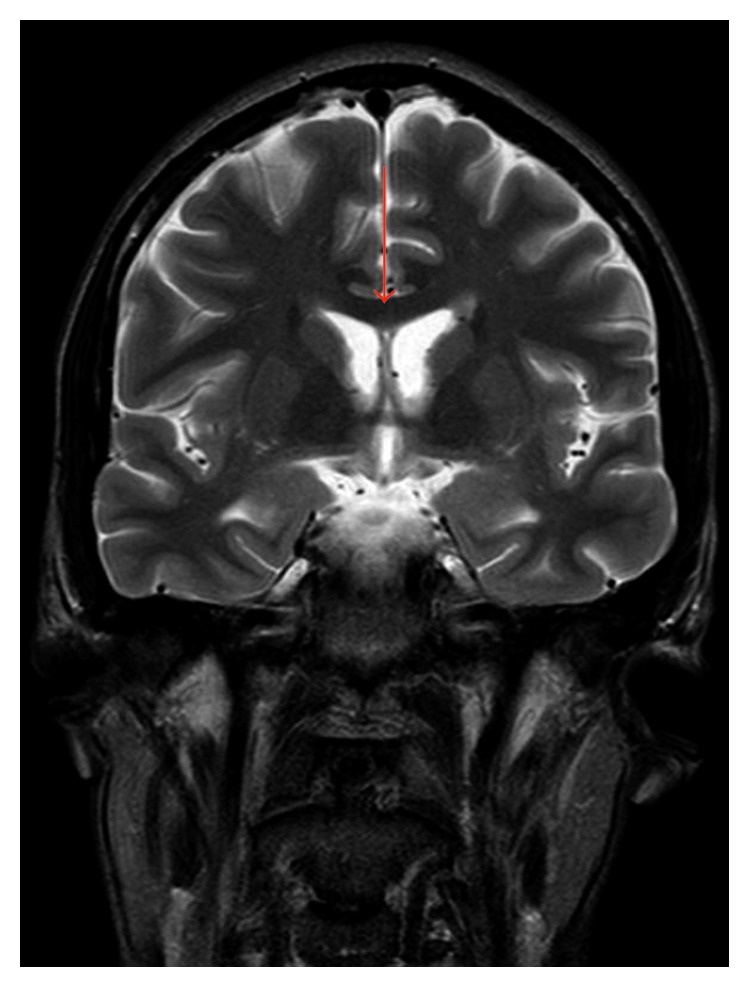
Ventricular hypersignal in cranial MRI (FLAIR), suggestive of ventriculitis.
